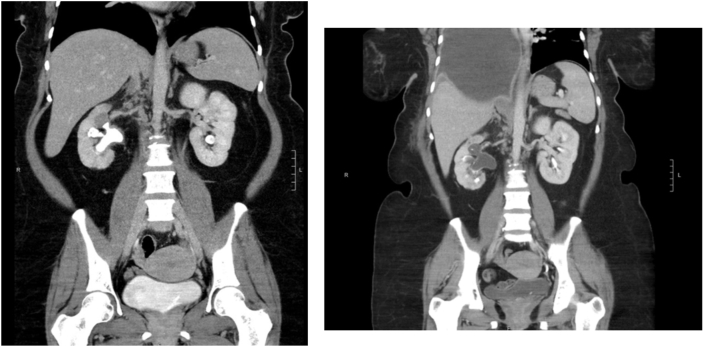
A large right staghorn calculus and moderate hydronephrosis at upper pole (A). Final CT scan with contrast showing minimal stone residuals on day of discharge (B).
